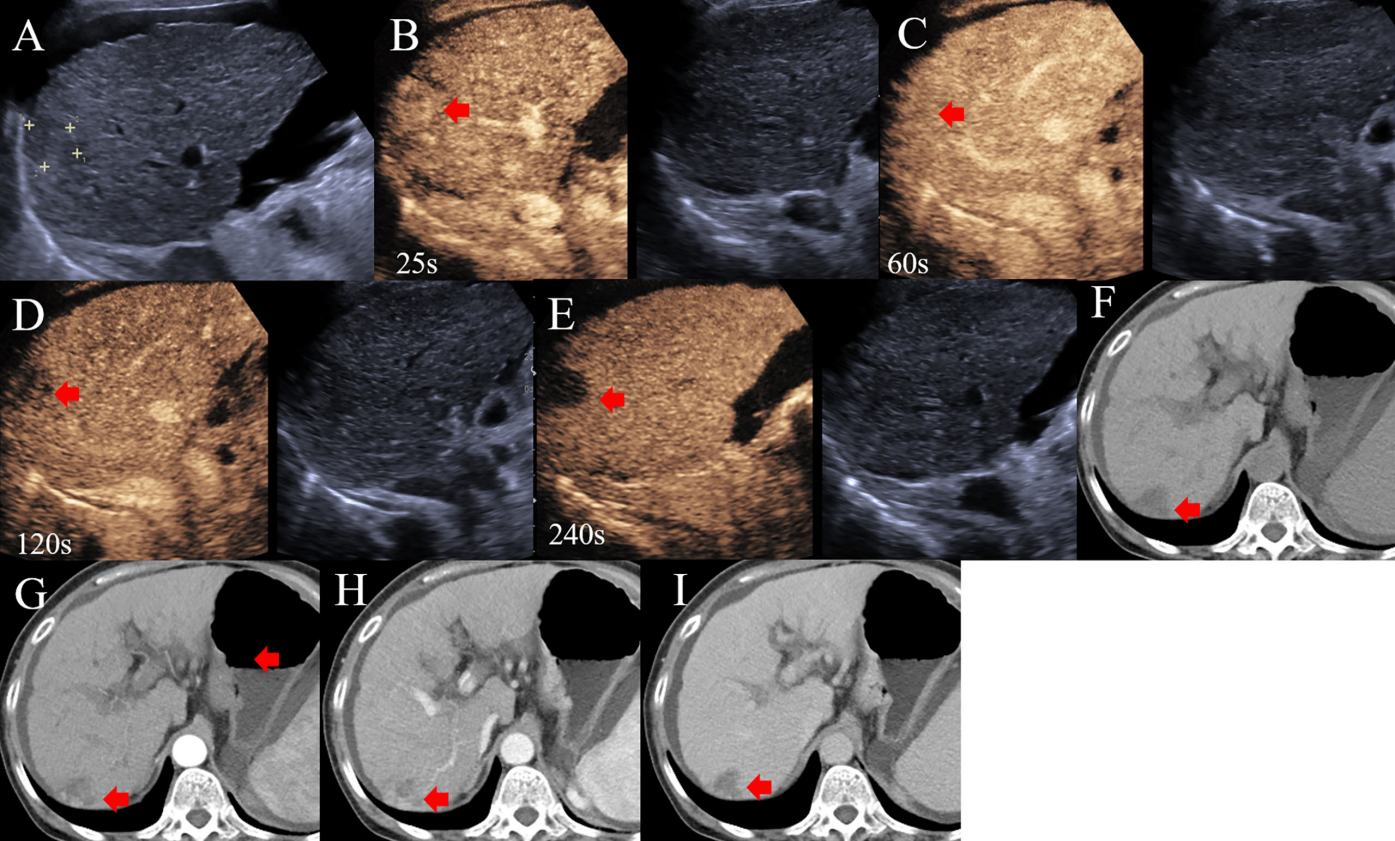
A 68-year-old woman with HBV related liver cirrhosis and elevated AFP (1210ng/ml) and CA19-9 (43.03 ng/ml). (A) A hypo-echoic lesion with a size of 1.9cm was observed by US. (B) The lesion showed APHE on CEUS; (C) without washout 1 min after injection of contrast agent; (D) Delay and mild washout was observed 2 min after injection; (E) The lesion appeared punched-out 4 min after injection of contrast agent. The lesion was categorized to CEUS LR-5. (F) A hypo-intensive lesion was found on CT; (G, H) The heterogeneous enhancement was observed on the arterial and portal phases of contrast enhanced CT; (I) The lesion was hypo-intensive on the delay phase and classified into CT LR-5. The final diagnosis was cHCC-CCAs, as confirmed by pathology.
